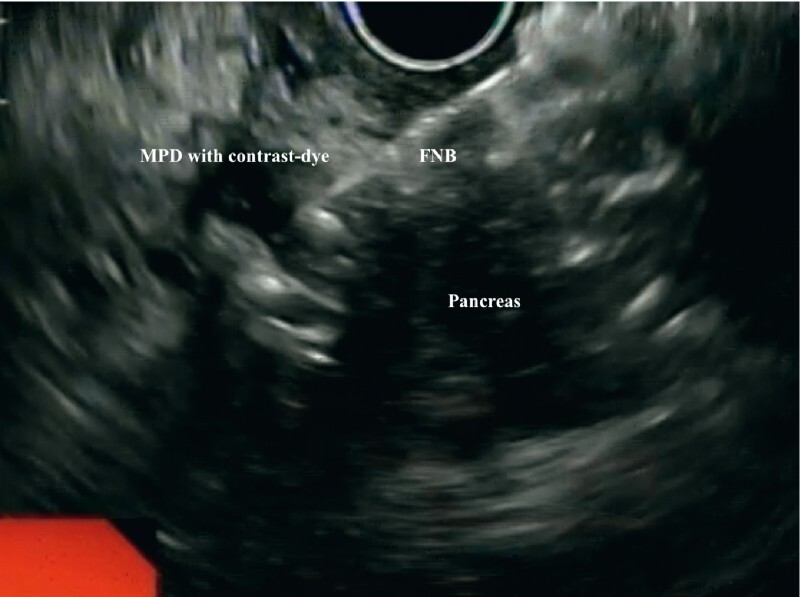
Endoscopic ultrasound (EUS) view of the transgastric EUS-guided insertion of a guidewire into the main pancreatic duct (MPD). FNB, fine-needle biopsy.
